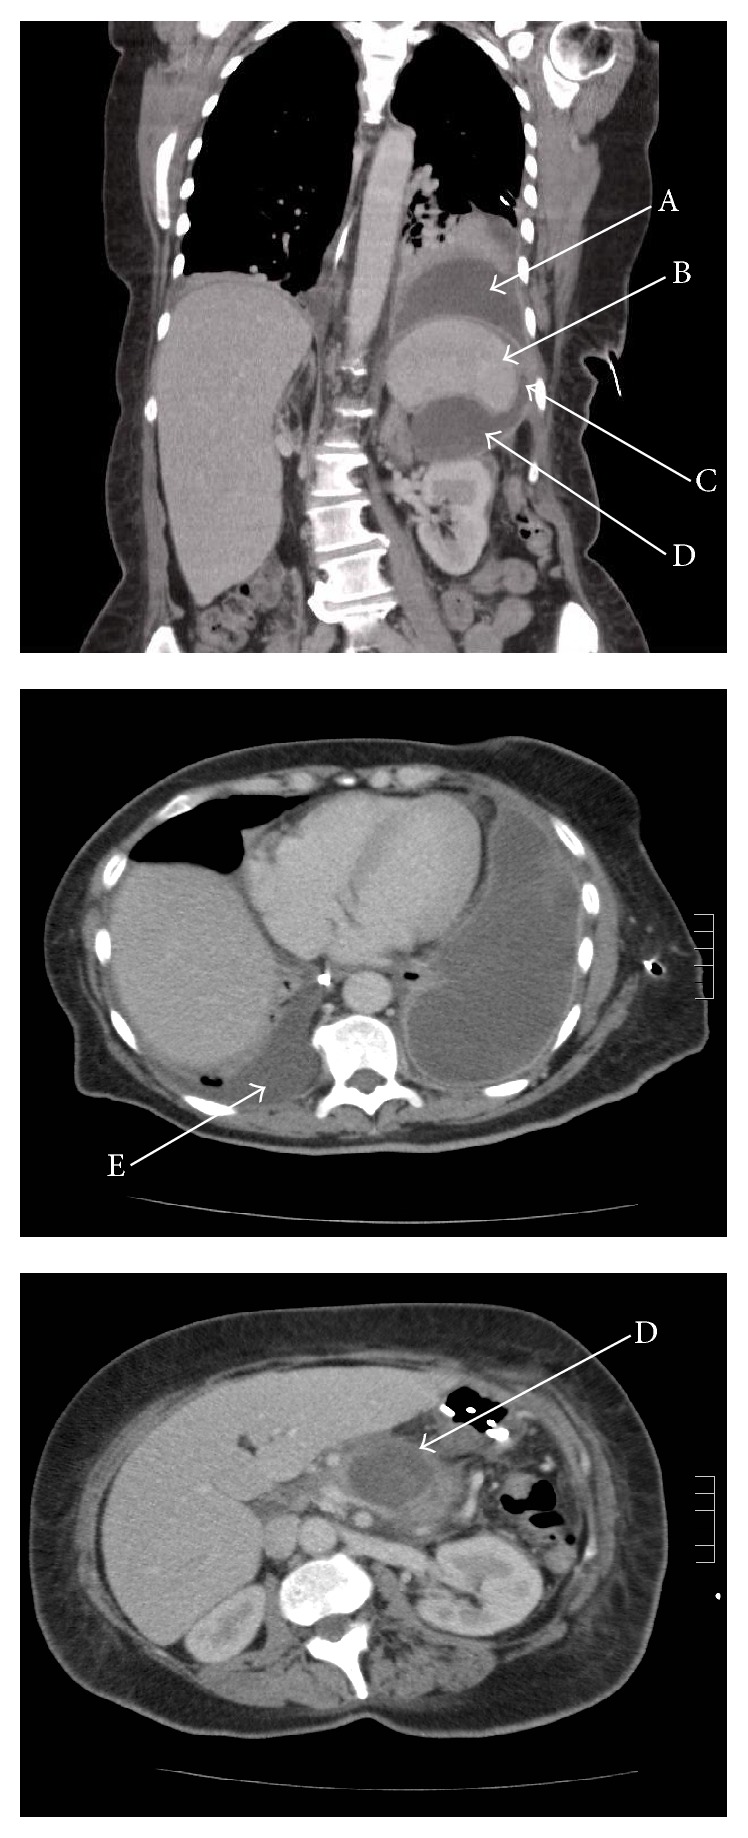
CT scan of the chest and abdomen demonstrating bilateral pleural effusions, the largest being in the left pleural cavity which is communicating directly with a pancreatic pseudocyst confirming the diagnosis of pancreatic pseudocyst-pleural fistula (PP-PF). Arrow A shows the position of the left pleural effusion communicating through fistula C with a large pancreatic pseudocyst D at the posterolateral aspect of the spleen B. Arrow E demonstrates the smaller right-sided pleural effusion.
